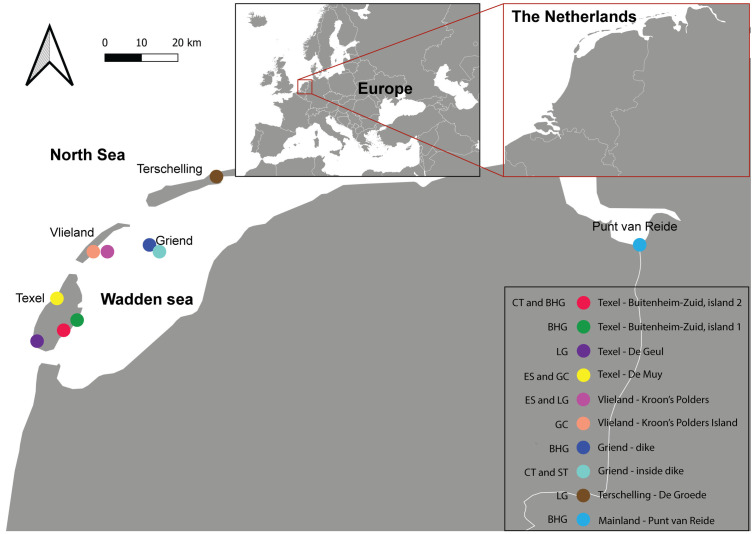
This figure indicates the location of The Netherlands within Europe; a map of The Netherlands; and the location of the colonies used in this study. The abbreviations of the species are as follows: CT: common tern; BHG: black-headed gull; LG: large gulls; ES: Eurasian spoonbill; GC: great cormorant; ST: Sandwich tern. Maps were made with Natural Earth. See S1 Table for more detailed information about each colony.
